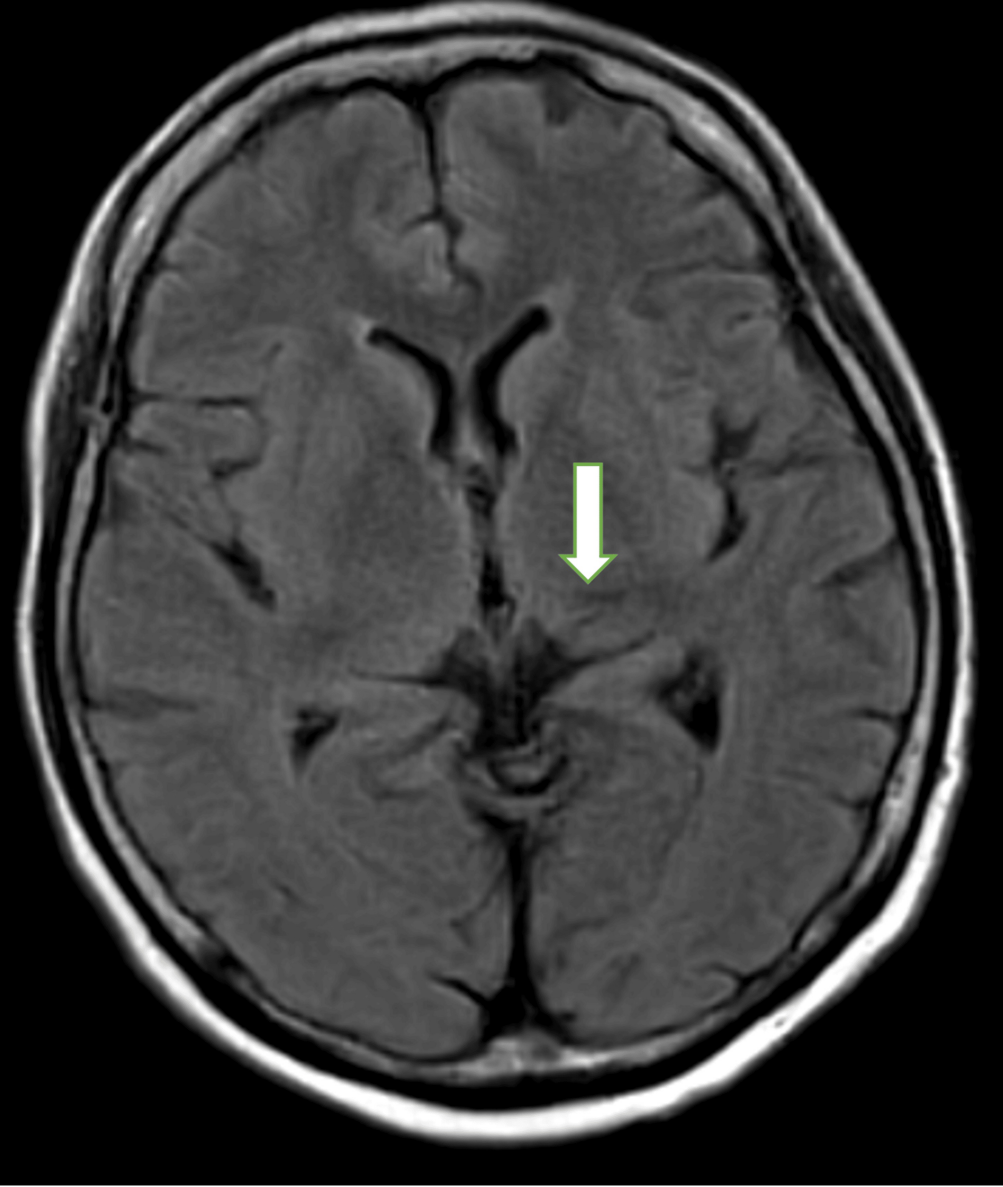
Head magnetic resonance imaging during the state of confusion. The arrow indicates hemosiderin deposition in the left thalamus, representing chronic changes from the previous cerebral hemorrhage.
